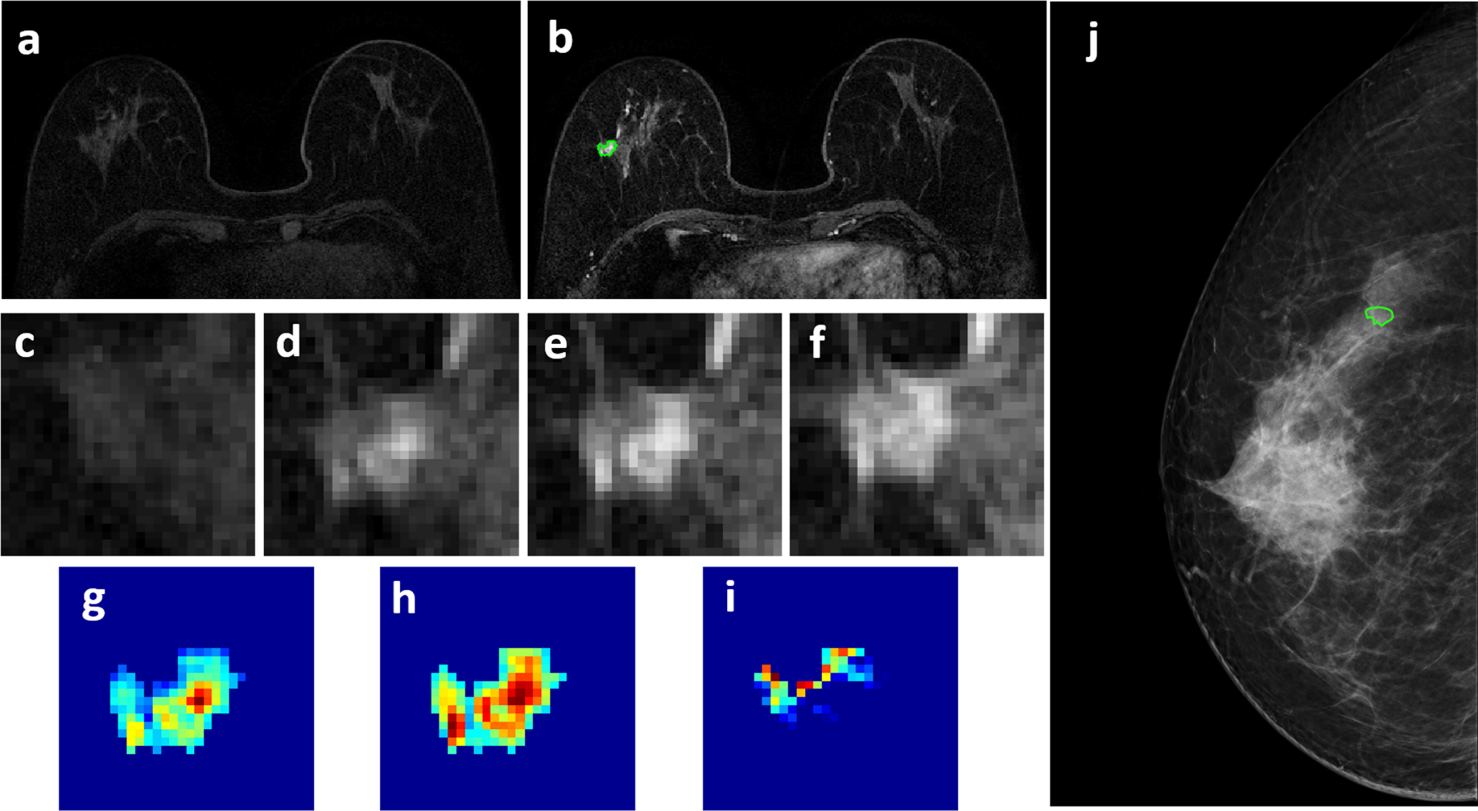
A 63-year-old patient with a 0.7 x 0.7 cm benign adenosis, showing a persistent DCE-MRI enhancement kinetics and determined as BI-RADS 3 on MRI. (A) F1 Pre-contrast image. (B) F2 post-contrast image. (C–I) Magnified images to demonstrate the margin and internal enhancements within the lesion. (C) F1 pre-contrast, (D) F2 post-contrast, (E) F3 post-contrast, (F) The last F6 post-contrast image. (G) The wash-in signal enhancement map F2-F1, (H) The F3-F1 signal enhancement map, (I) The wash-out F6-F3 map. (J) The lesion is not seen on mammography, determined as BI-RADS 2, and an area is outlined with the guidance of MRI. The probability predicted by MRI, mammography, and combined radiomics models were: 0.42, 0.44, 0.15, respectively, true negative.
